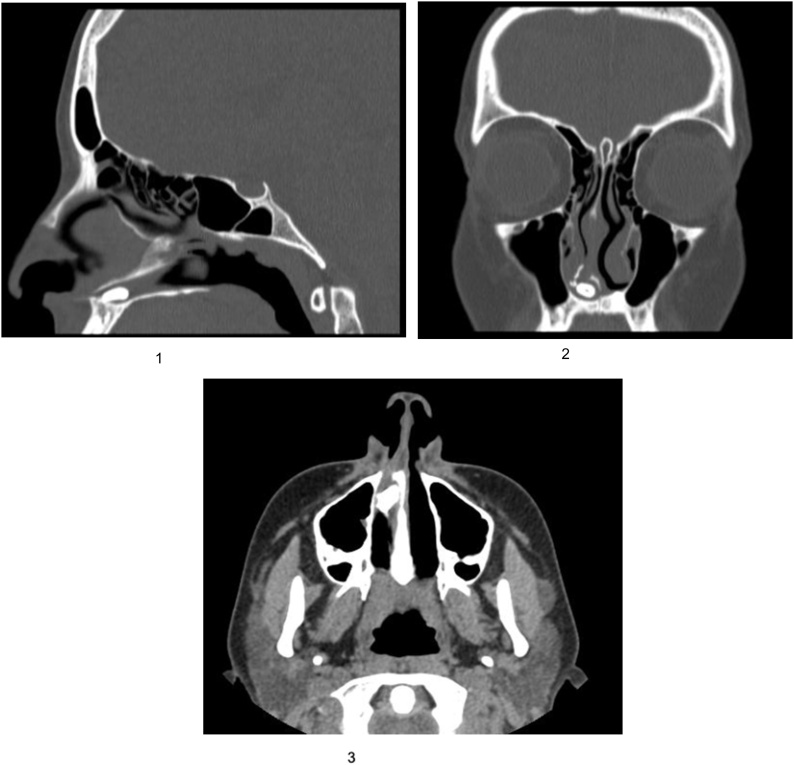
(1) Sagittal View CT-scan. (2) Coronal View CT-scan. (3) Axial View CT-Scan.
